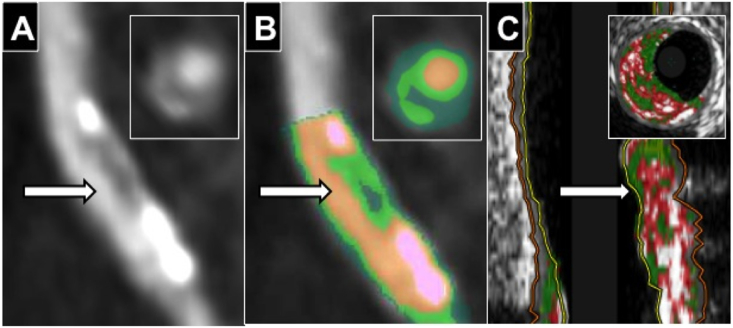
Coronary CTA Napkin-Ring sign and corresponding plaque map. (A) ‘Napkin-Ring’ sign with low attenuation plaque surrounding by higher attenuation non-calcified plaque. (B) Plaque Map with plaque quantification necrotic core (35.3%-dark green) surrounded by fibrous plaque (51.5%-light green)(orange = lumen, calcified plaque = pink (13.2%) Necrotic core/fibrous plaque ratio = 0.69. (C) Co-registered VH-IVUS fibroatheroma (red = necrotic core, green = fibrous plaque and white = calcified plaque). Insets show cross-sectional images at arrows.
